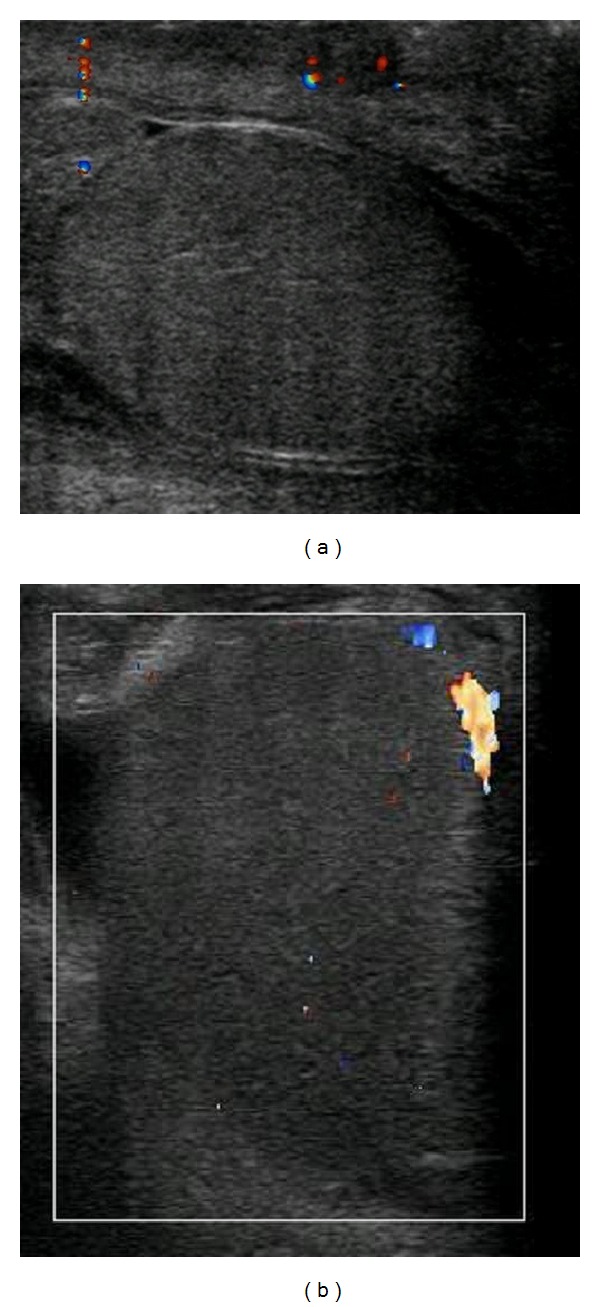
Scrotal ultrasound images with color Doppler showing decreased blood flow to the left testicle (a) and right testicle (b). Both images are in the sagittal view.
